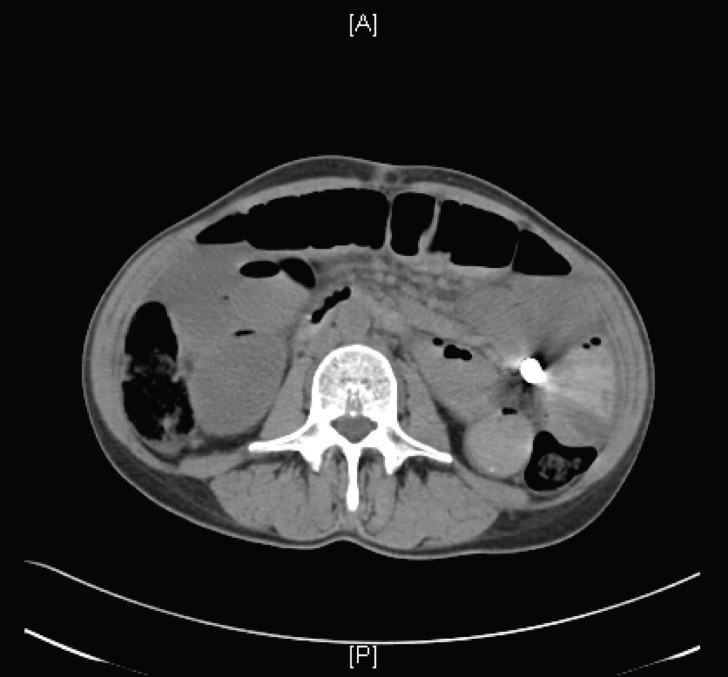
Abdominal CT demonstrating a retained capsule in the mid-portion of the jejunum.
